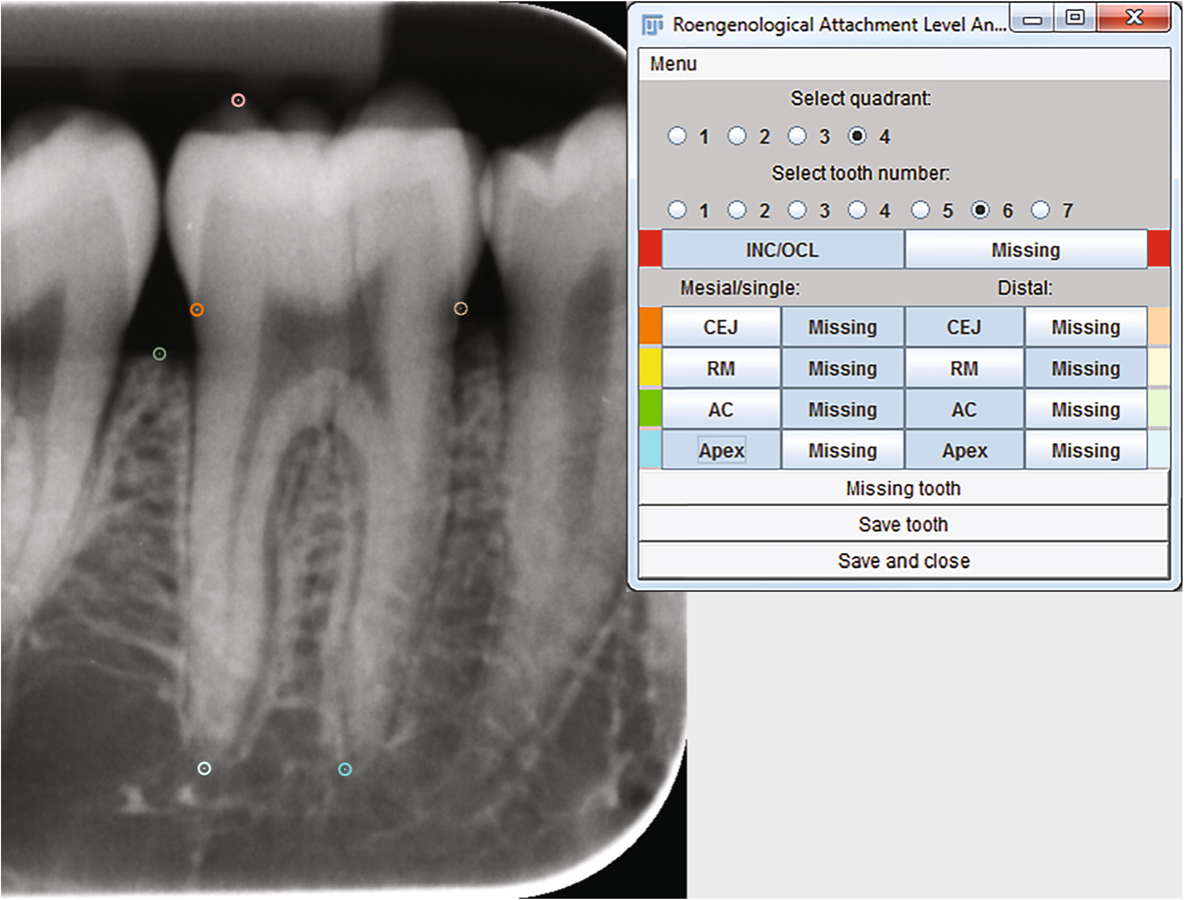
Radiograph with markings and application (plugin) running
